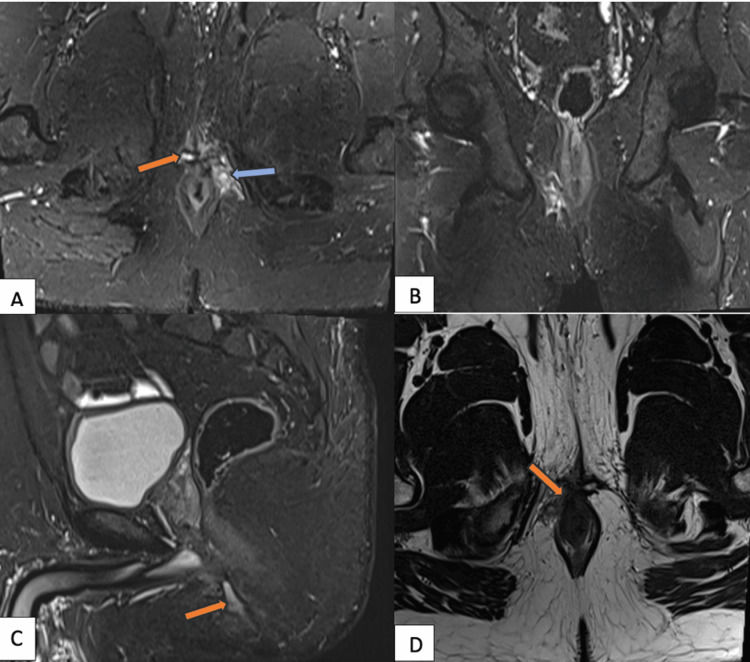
MRI fistulogram demonstrating a St. James grade 4 perianal fistula with secondary ramifications and abscess formation MRI: magnetic resonance imaging; STIR: short tau inversion recovery STIR coronal image (A) shows a transsphincteric fistulous tract (orange arrow) with an associated abscess cavity (blue arrow) in the ischioanal fossa. Coronal (B) and sagittal (C) STIR images reveal multiple ramifications extending into adjacent soft tissues. Axial T2-weighted image (D) further delineates the complex tract traversing both sphincters (orange arrow)
